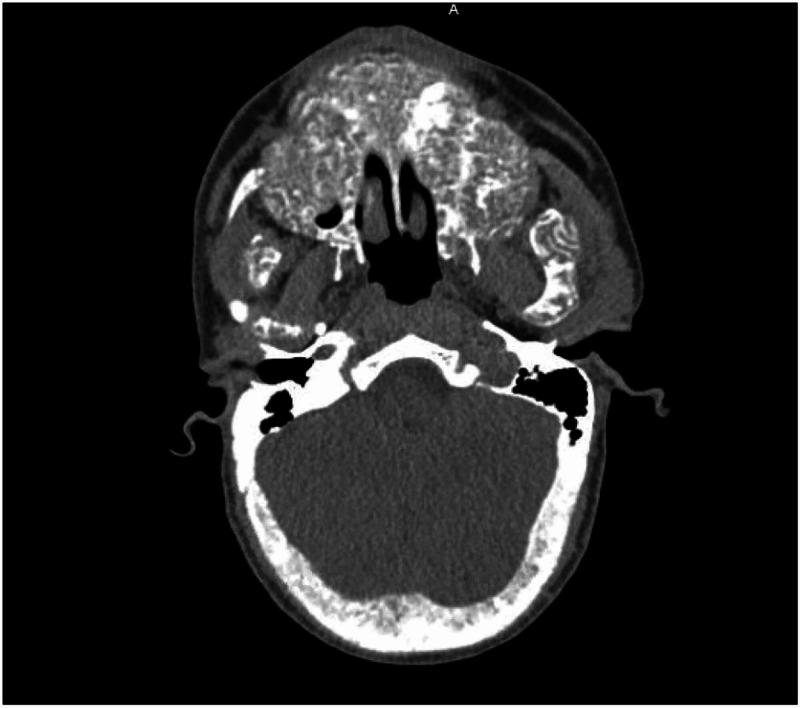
Axial view - Non-contrast CT showing expanded mandible with prominent dense trabeculae. Involvement of alveolar margin is also noted, with worse findings on the left side.
